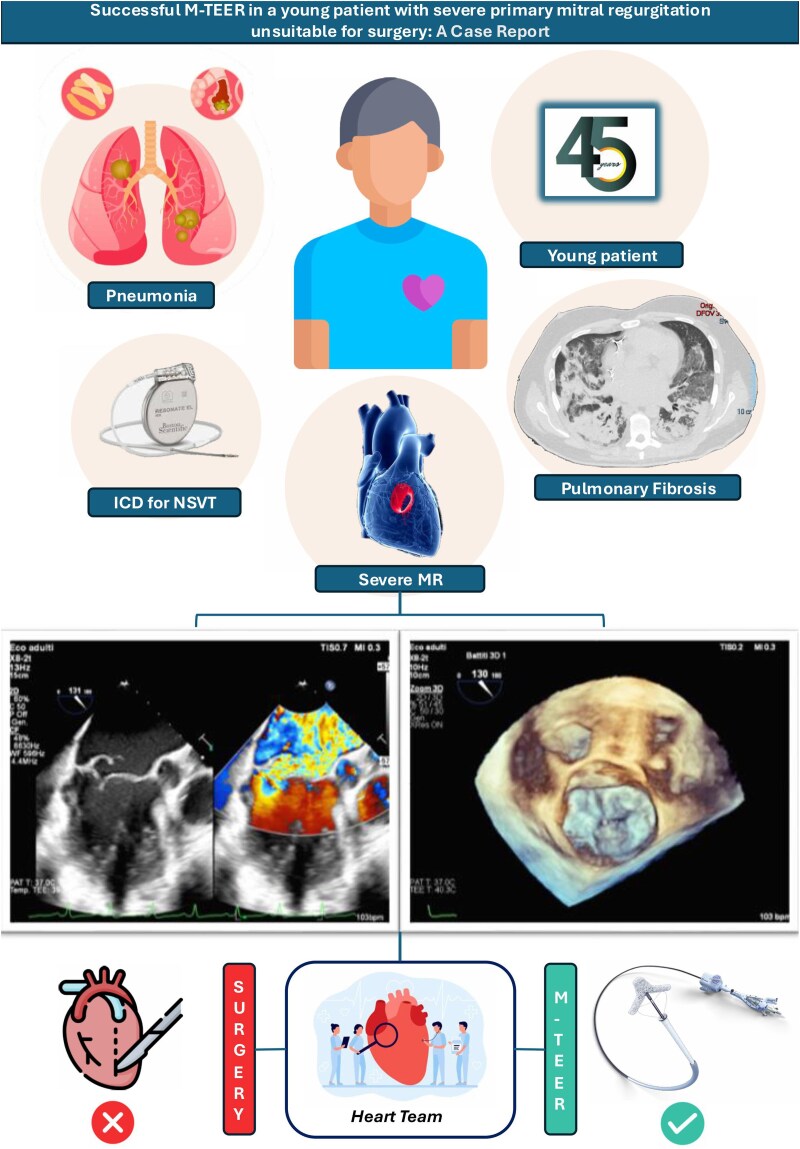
Patient history, risk factor, and Heart Team decision for M-TEER; pre-procedural transoesophageal echocardiography images including LVOT views and 3D images; M-TEER, transcatheter edge-to-edge mitral valve repair.
